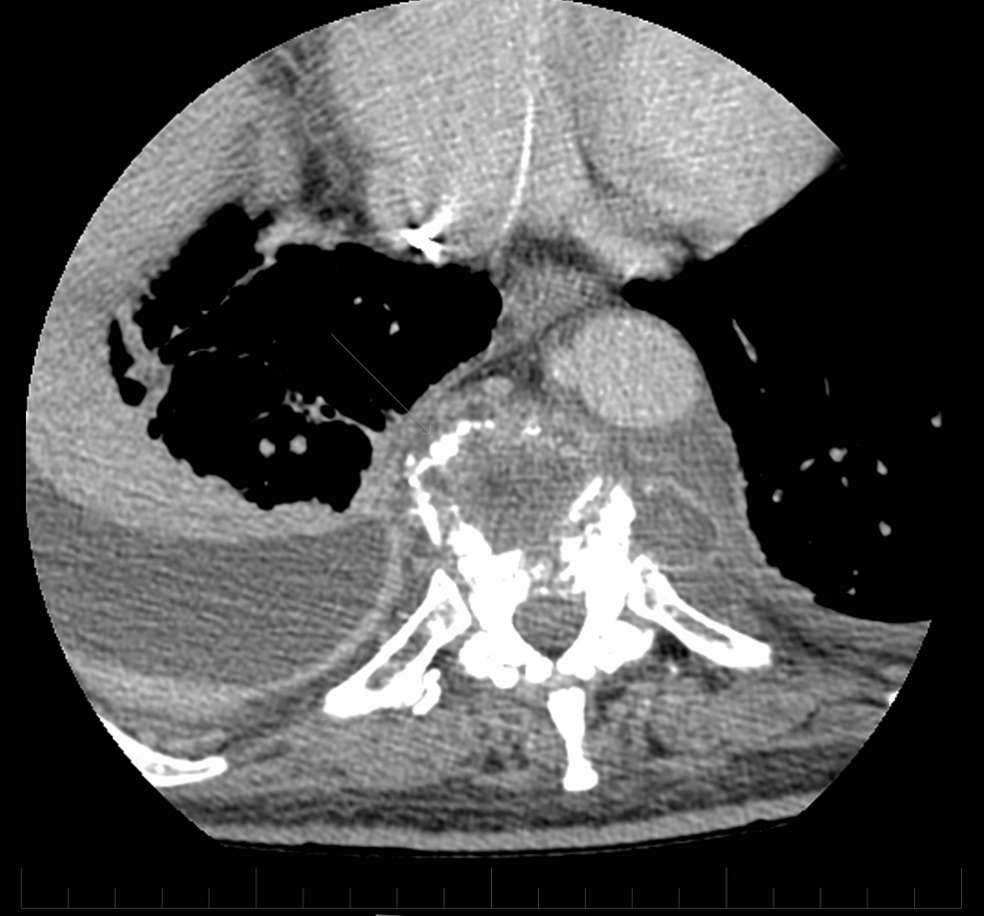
Computed tomography axial view of the thoracic spine during subsequent admission This demonstrates significant worsening bony destruction of the T9-T10 vertebral bodies involving the adjacent endplates. There is additional prevertebral soft tissue edema.
